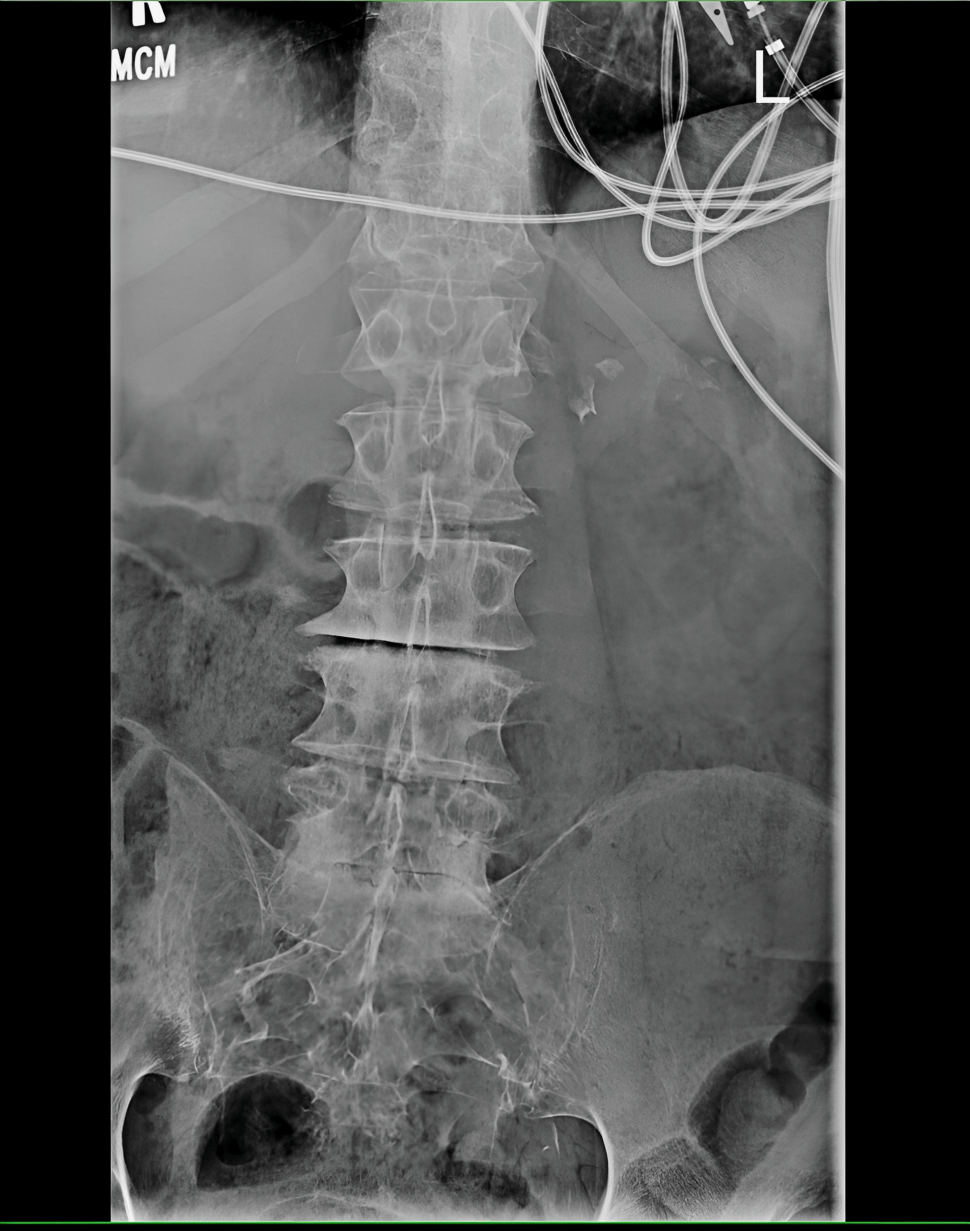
The lumbar spine X-ray demonstrates multilevel degenerative disc disease and facet arthrosis, most severe in the lower lumbar spine. No compression deformity or malalignment is observed.
